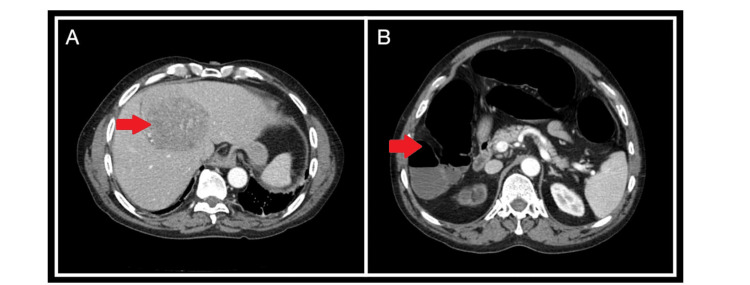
CT abdomen CT of the abdomen showing the liver lesion (A) and dilated loops of the bowel (B).
